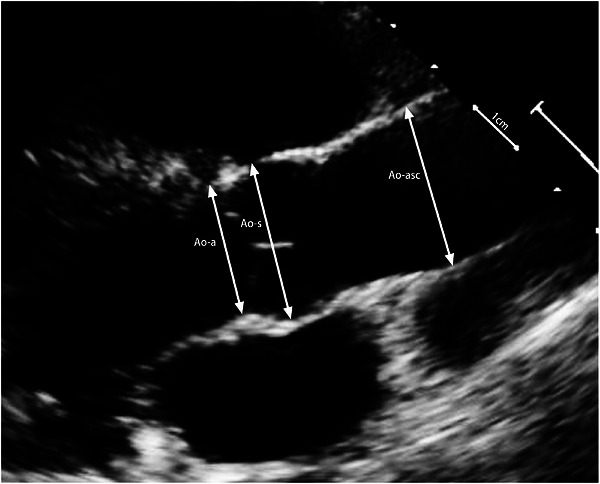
From the parasternal long‐axis view, aortic root diameters (annulus, sinuses of Valsalva, and proximal ascending aorta) were measured at end‐diastole using a leading‐edge to leading‐edge method.
